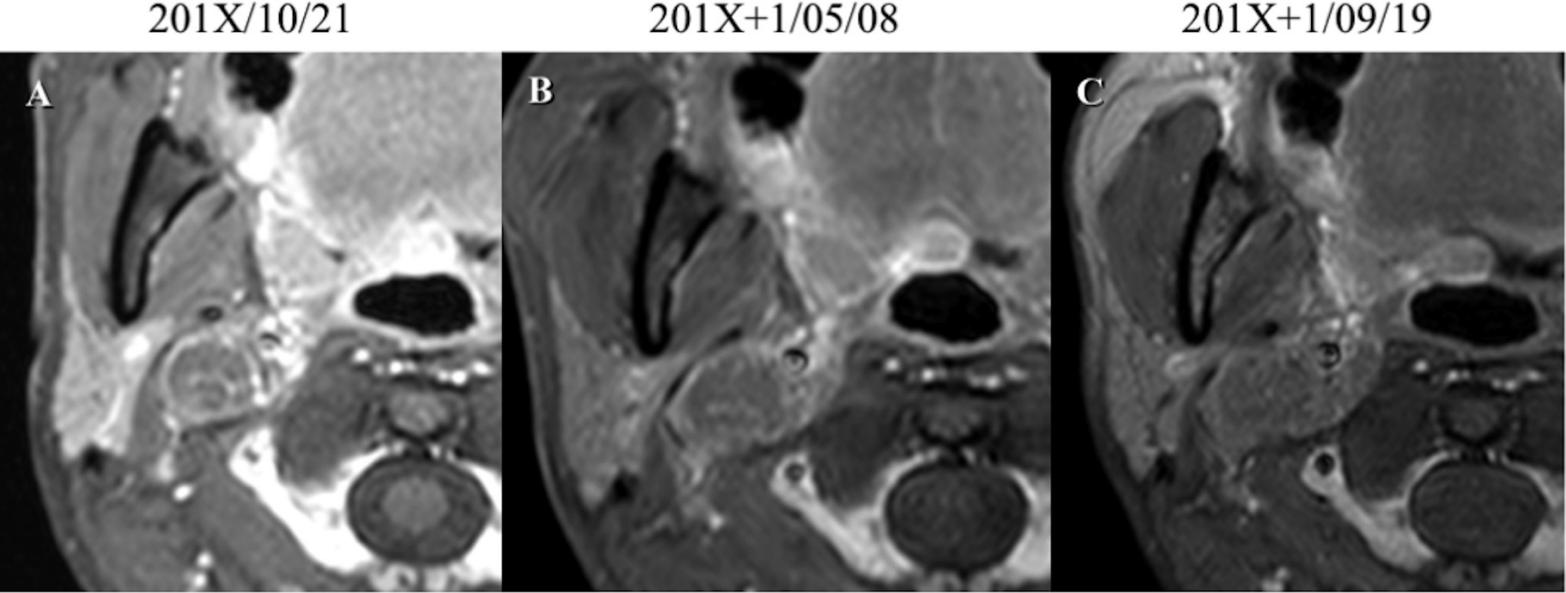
Tumor in the jugular foramen extending into the deep cervical region (Type C jugular foramen tumor). The tumor did not exhibit internal flow voids. It exhibited hypointensity on T1-weighted MRI, and iso-to hyperintensity on T2-weighted MRI. Furthermore, contrast-enhanced T1 MRI showed that the tumor rim was clearly enhanced (A). On a contrast-enhanced CT scan, this tumor displayed slightly heterogenous enhancement. Tumor had grown rapidly (B) and started to surround the internal carotid artery (C).
